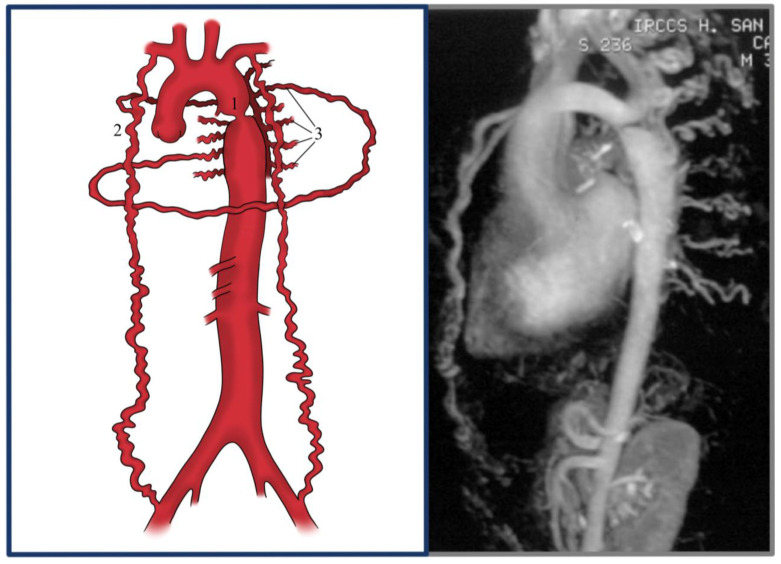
Localized CoA (1) with the internal thoracic artery (2) and intercostal (3) hypertrophy: graphical presentation and 3D MRI.
